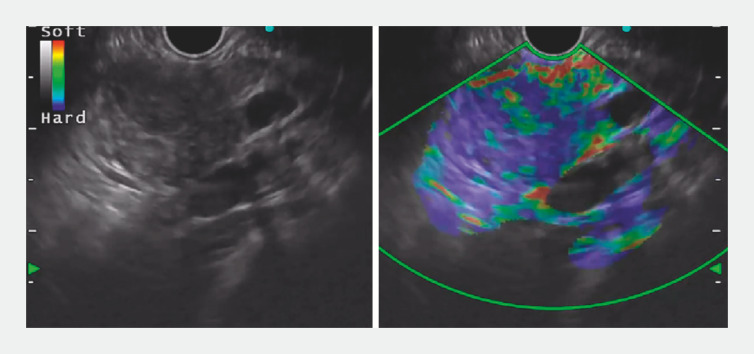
Endoscopic ultrasound elastography revealed a 2 × 2.5-cm hypoechoic mass that was predominantly hard (blue) with dispersed heterogeneous soft (green) areas.
